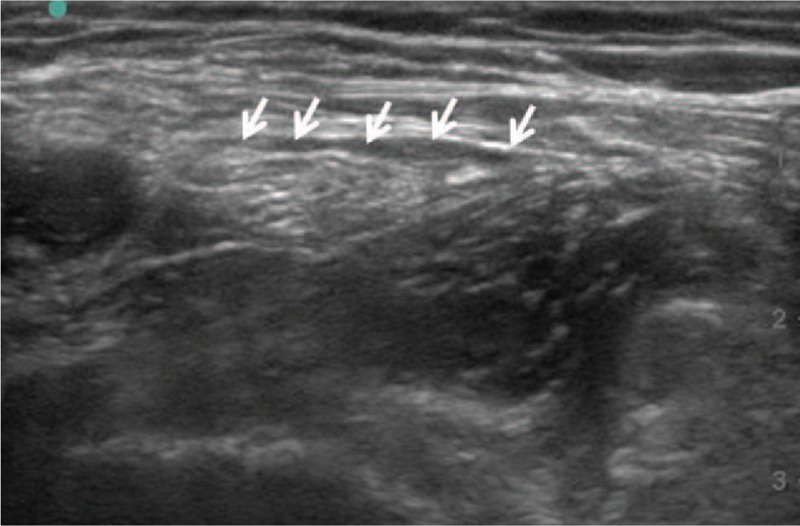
Ultrasound image of the femoral nerve. The local anesthetic was deposited between the fascia iliac and femoral nerve (arrows).
